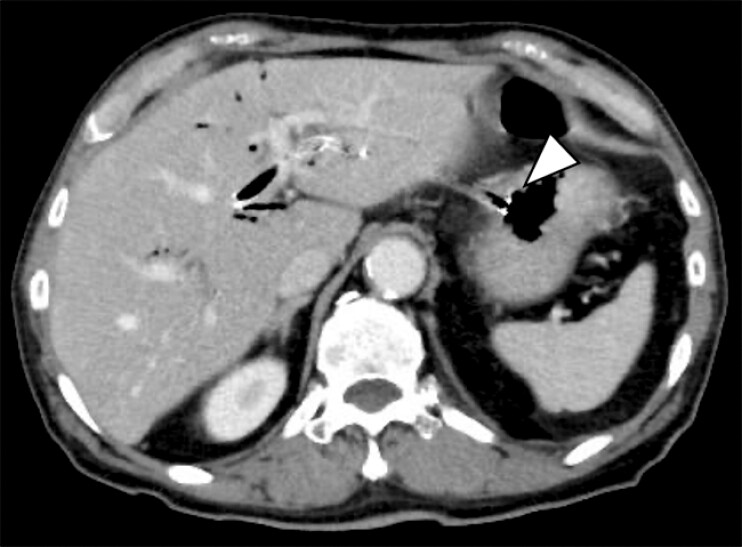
Computed tomography on the day after EUS-HGS reveals that the gastric side of the SEMS is partially dislodged toward the abdominal cavity (arrowhead).
